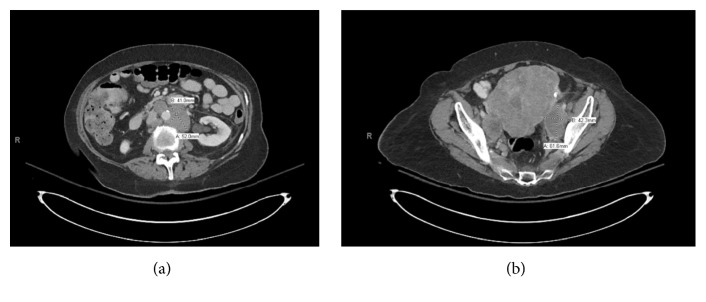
CT abdomen and pelvis: (a) bulky retroperitoneal adenopathy of the abdomen as well as bilateral iliac chain adenopathy. (b) Enlargement and nodularity of the uterus are seen, worrisome for diffusely infiltrative carcinoma.
